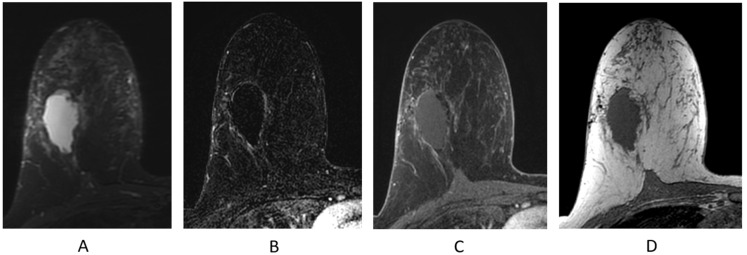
Seroma/surgical cavity on breast MRI. (A) Axial STIR sequence; (B) axial post-contrast subtraction; (C) axial post-contrast T1-weighted fat-suppressed sequence; (D) axial T1-weighted non-fat-suppressed sequence.
